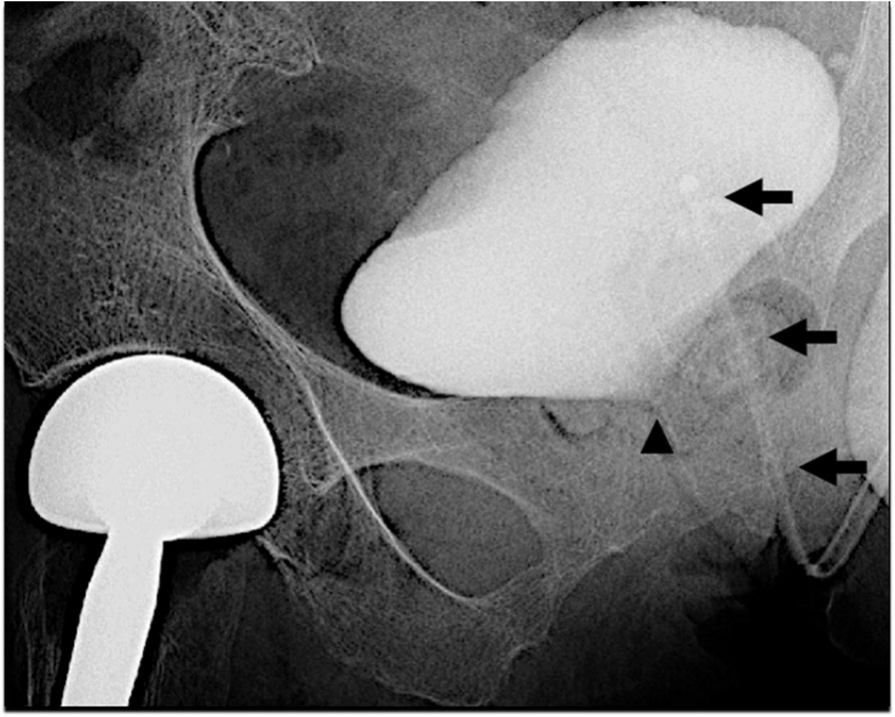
Postoperative right hip radiograph. Lauenstein projection showed a urethral catheter (arrow) not passing through the internal urethral orifice (arrowhead) with retained urine containing contrast medium in the bladder
